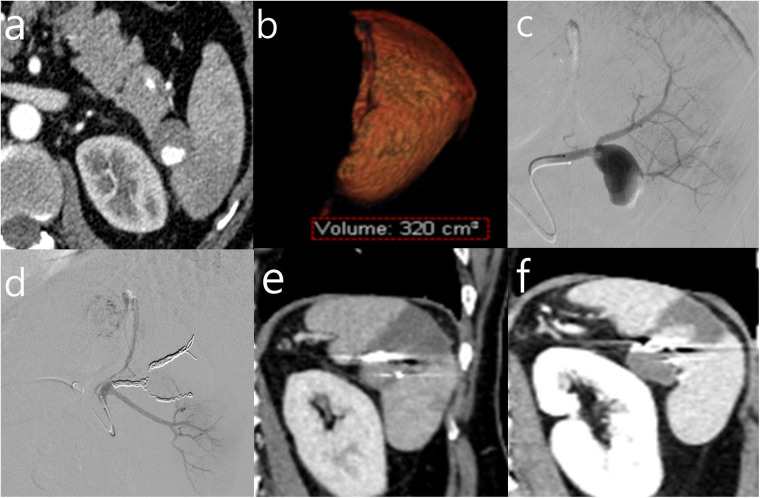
Panel a shows a CT scan revealing a saccular aneurysm at the splenic hilum measuring 23.3 mm. Panel b displays a 3D volumetric rendering of the spleen with a labeled volume of 320 cm³. Panel c shows an angiographic image with the hilar aneurysm and two distal branches. Panel d shows selective embolization of the proximal and distal branches with microcoils. Panel e is a follow-up CT seven days post-embolization showing splenic infarction involving approximately 62.5% of the spleen with a volume of 218 mL. Panel f is a follow-up CT at 25 days showing partial splenic regeneration with an increased volume of 243 mL
